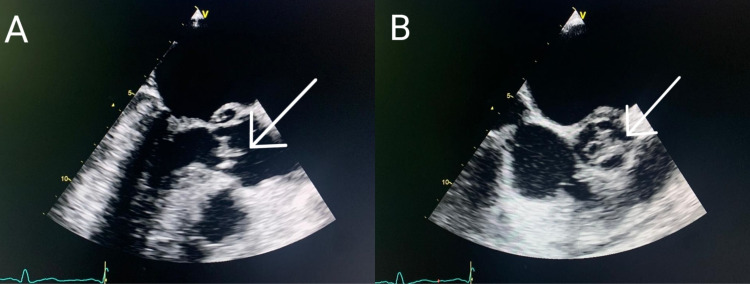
Transesophageal echocardiogram. A: vegetation at the mitral valve. B: vegetation at the aortic valve.
